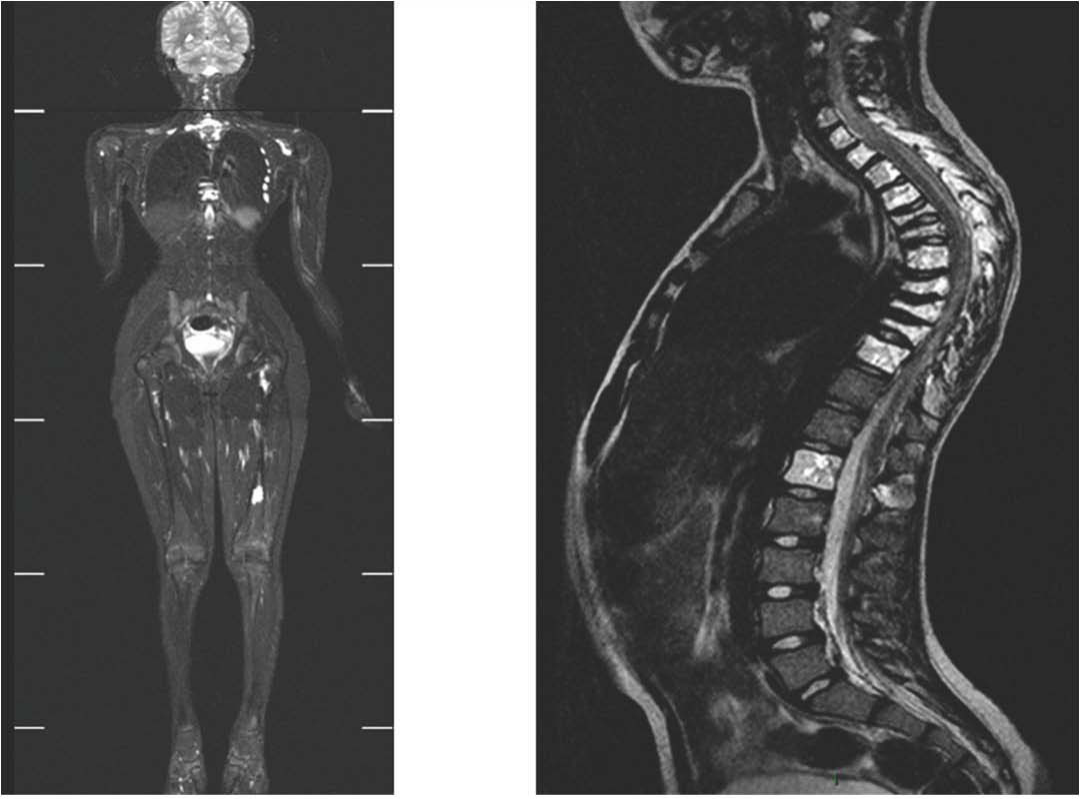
Whole body coronal and sagittal MRI (case #20). T2-weighted MRI of spinal (C2; C3; C6→T10; L1), costal, left scapular and left femoral lesions.
